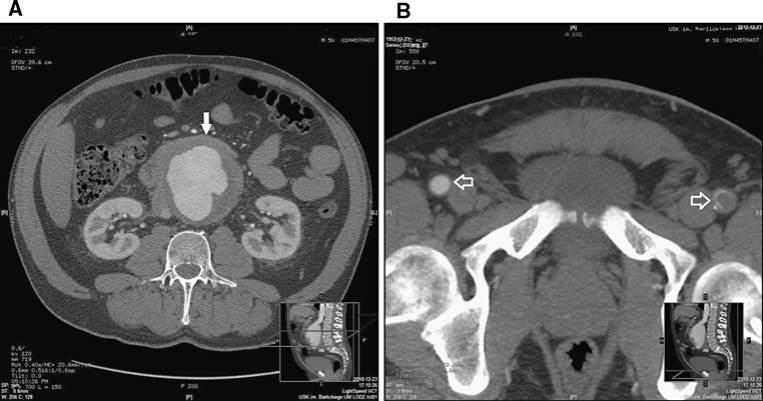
Angio-CT axial scan, aortic aneurysm below the level of the renal arteries, with irregular adhering thrombus. A Within the anterior aortic wall there is a narrow, irregular soft tissue infiltration that suggests the presence of inflammation (mantle core sign) (*arrow*). B Axial scan at the level of femoral arteries (*arrows*) reveals an embolism of the left artery. The right femoral artery is patent
